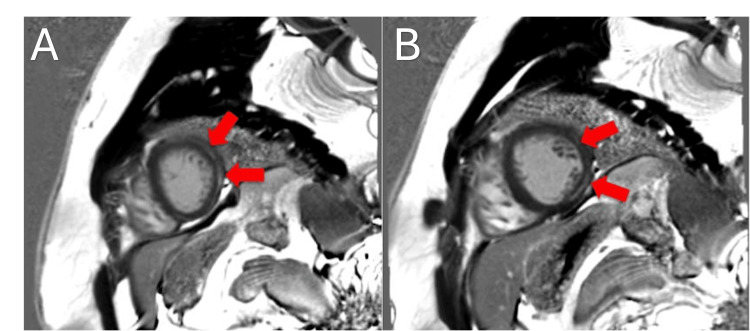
Cardiac MRI (CMRI) obtained during hospitalization. Panels (A) and (B) represent short-axis CMRI slices at different levels. Red arrows indicate increased T2 signal in the mid-anterolateral and inferolateral walls corresponding with areas of subepicardial delayed enhancement.
